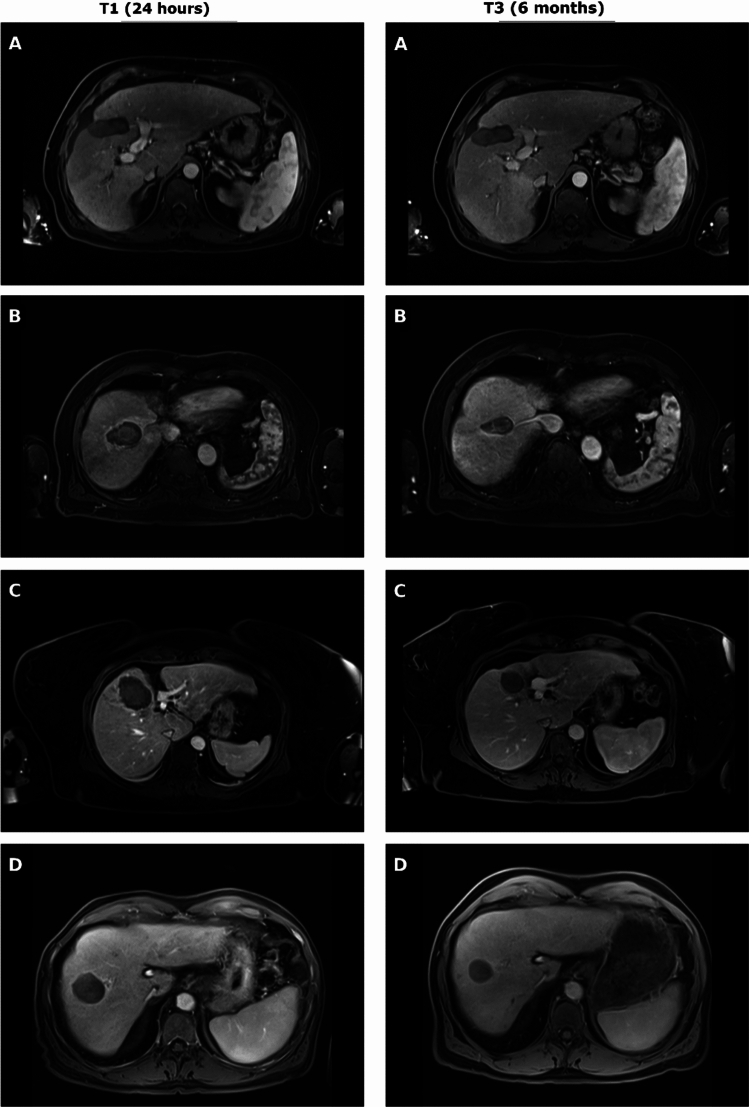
Representative MRI cases after TACE + MWA. A Case A, male, 77 years, HCC, high-power protocol: T1 shows complete coverage; at T3 contraction is moderate with preserved margins. B Case B, male, 66 years, HCC, high-power protocol: complete necrosis at T1; mild shrinkage at T3 with stable margins. C Case C, female, 79 years, breast cancer liver metastasis, low-power protocol: sharply demarcated ablation zone at T1; pronounced contraction by T3 with narrowing of the rim. D Case D, male, 68 years, HCC, high-power protocol: broad ablation zone at T1; limited contraction at T3 with margin integrity preserved
